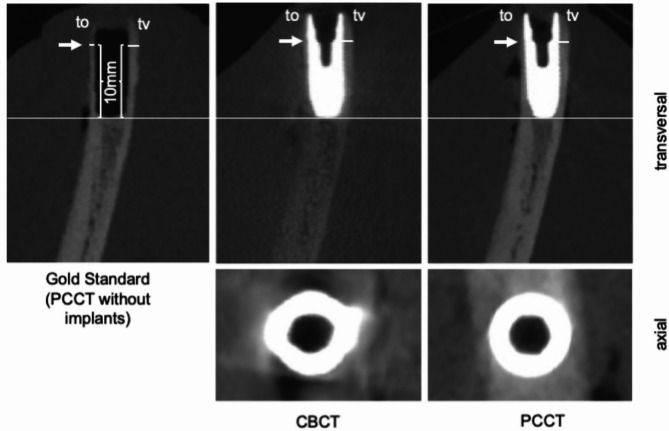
Representation of the measurement systematics and the different imaging techniques in PCCT and CBCT. The images are registered to one another. The subjectively better image quality of the PCCT scans is clearly visible. Furthermore, the oral bone lamella (to) can be identified in both the gold standard and the PCCT images with implants at the same location (indicated by the arrow). In contrast, in the CBCT image, this is not assessable or visible at the same location due to the artifacts generated. This is also visible in the axial slices. The lower level of artifacts in the PCCT also allows for the visualization of individual implant threads, as well as precise measurement of the vestibular bone thickness (tv)
